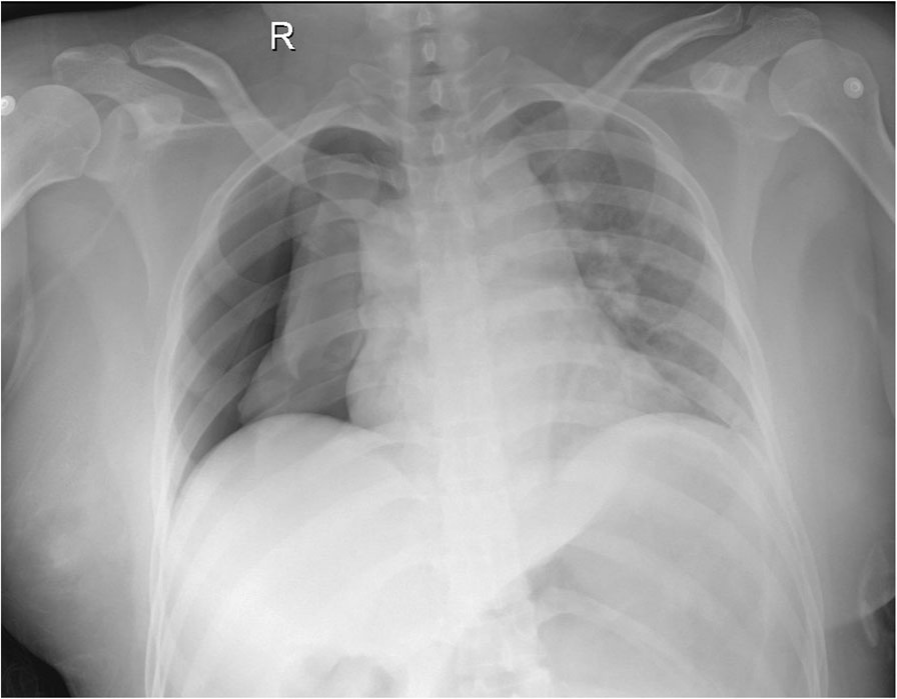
Chest X-ray in the operating room
